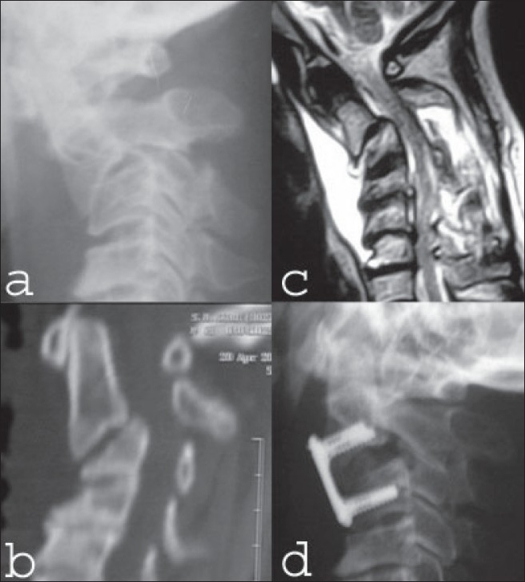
62 years old gentleman presented with C2-C3 bifacetal dislocation three weeks after the injury. Lateral x-rays (a) reconstructed sagittal CT scan (b) shows C2-C3 facetal dislocation. Mid sagittal T2WI of MRI (c) shows C2-C3 subluxation with cord compression. The patieints was treated skull traction to achieve partial reduction. Later on anterior C2-3 discectomy, fusion and fixation with locking plate and screw was done. Post operative lateral x-ray (d) shows reduction, C2-3 fusion and plate application
